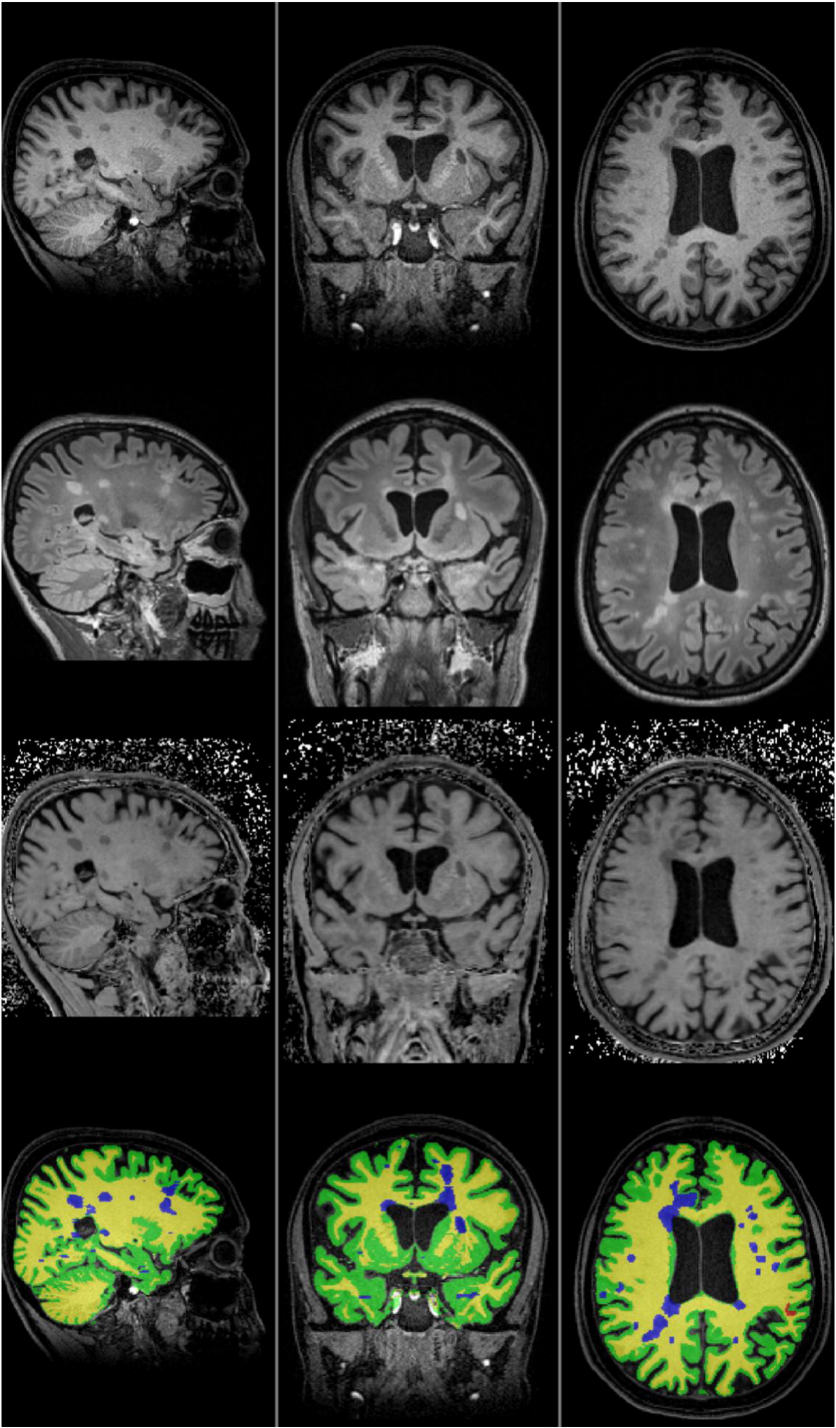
Representative images from a subject with a moderate lesion load. MPRAGE (top row), T2-w FLAIR (second row), resulting MTR map (third row), and ROI classifications overlaid on the MPRAGE (bottom row). Yellow = NAWM, green = NAGM, blue = WML, red = GML.
